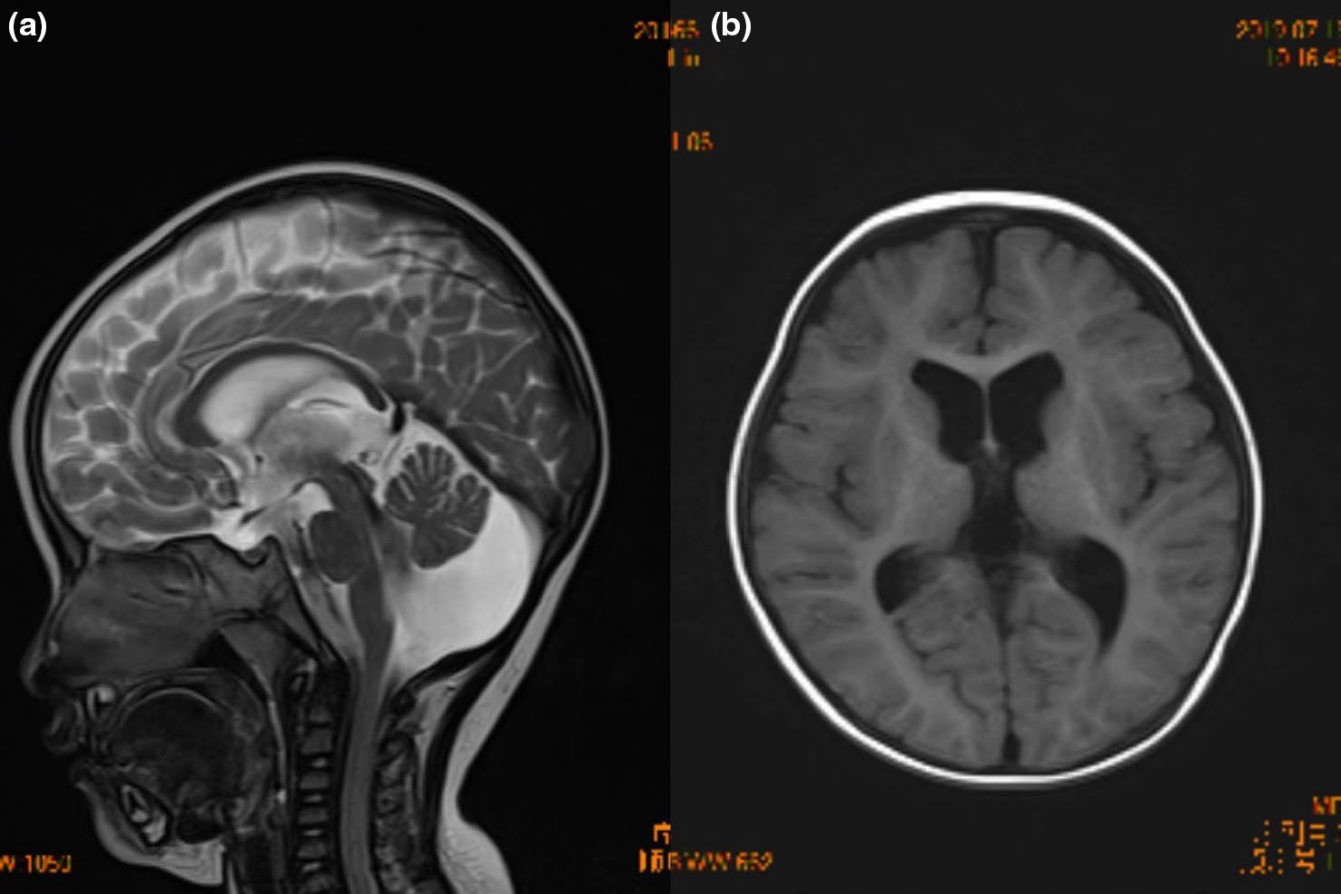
The brain magnetic resonance imaging (MRI) of the patient. The bilateral ventricles and the third ventricle were widened, (a) the midbrain aqueduct was not clear, (b) the lateral fissure cistern on both sides was slightly widened, the left temporal base extracerebral space was widened, and mega cisterna magna was found.
